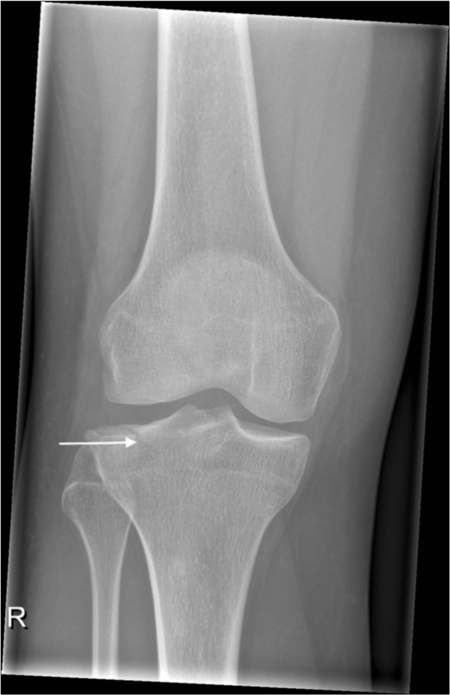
Anteroposterior X-ray view of a tibial plateau fracture (Schatzker II) with a white arrow indicating the upper part of the fracture, which led to a split, depression > 2 mm and loss of convexity of the lateral tibial plateau
